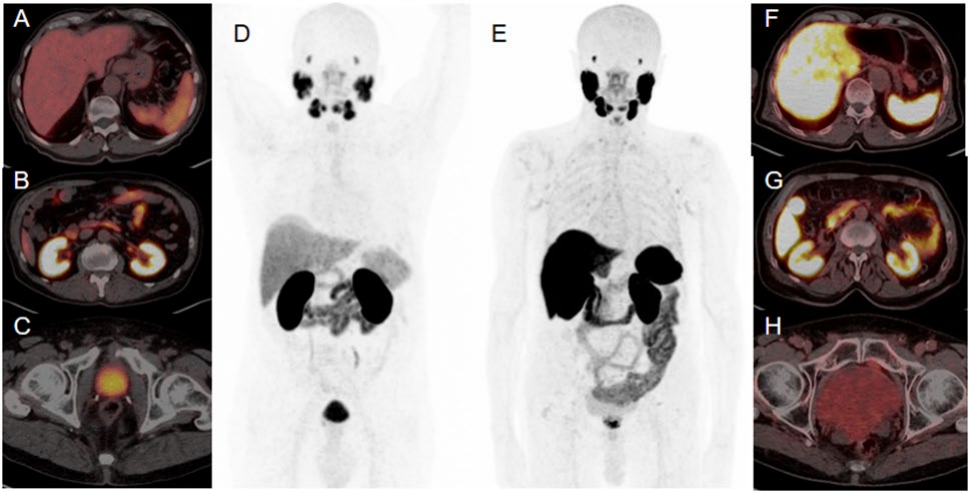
Physiological distribution of [^68^Ga]-PSMA11 PET/CT (A–D) and [^18^F]-PSMA-1007 PET/CT (E–H). [^18^F]- PSMA-1007 has a lower urinary clearance in comparison to [^68^Ga]-PSMA11 (C vs. H), but a higher hepatic elimination (A vs. F)
